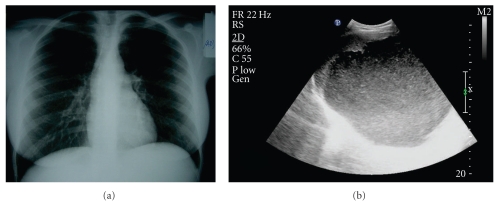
(a, b) Plain chest X-ray showing the elevation of the left hemidiaphragm (a). Abdominal ultrasound (US) showing a large, round hypoechoic lesion with internal echoes and regular thin wall surrounded by a peripheral rim of splenic tissue (b).
